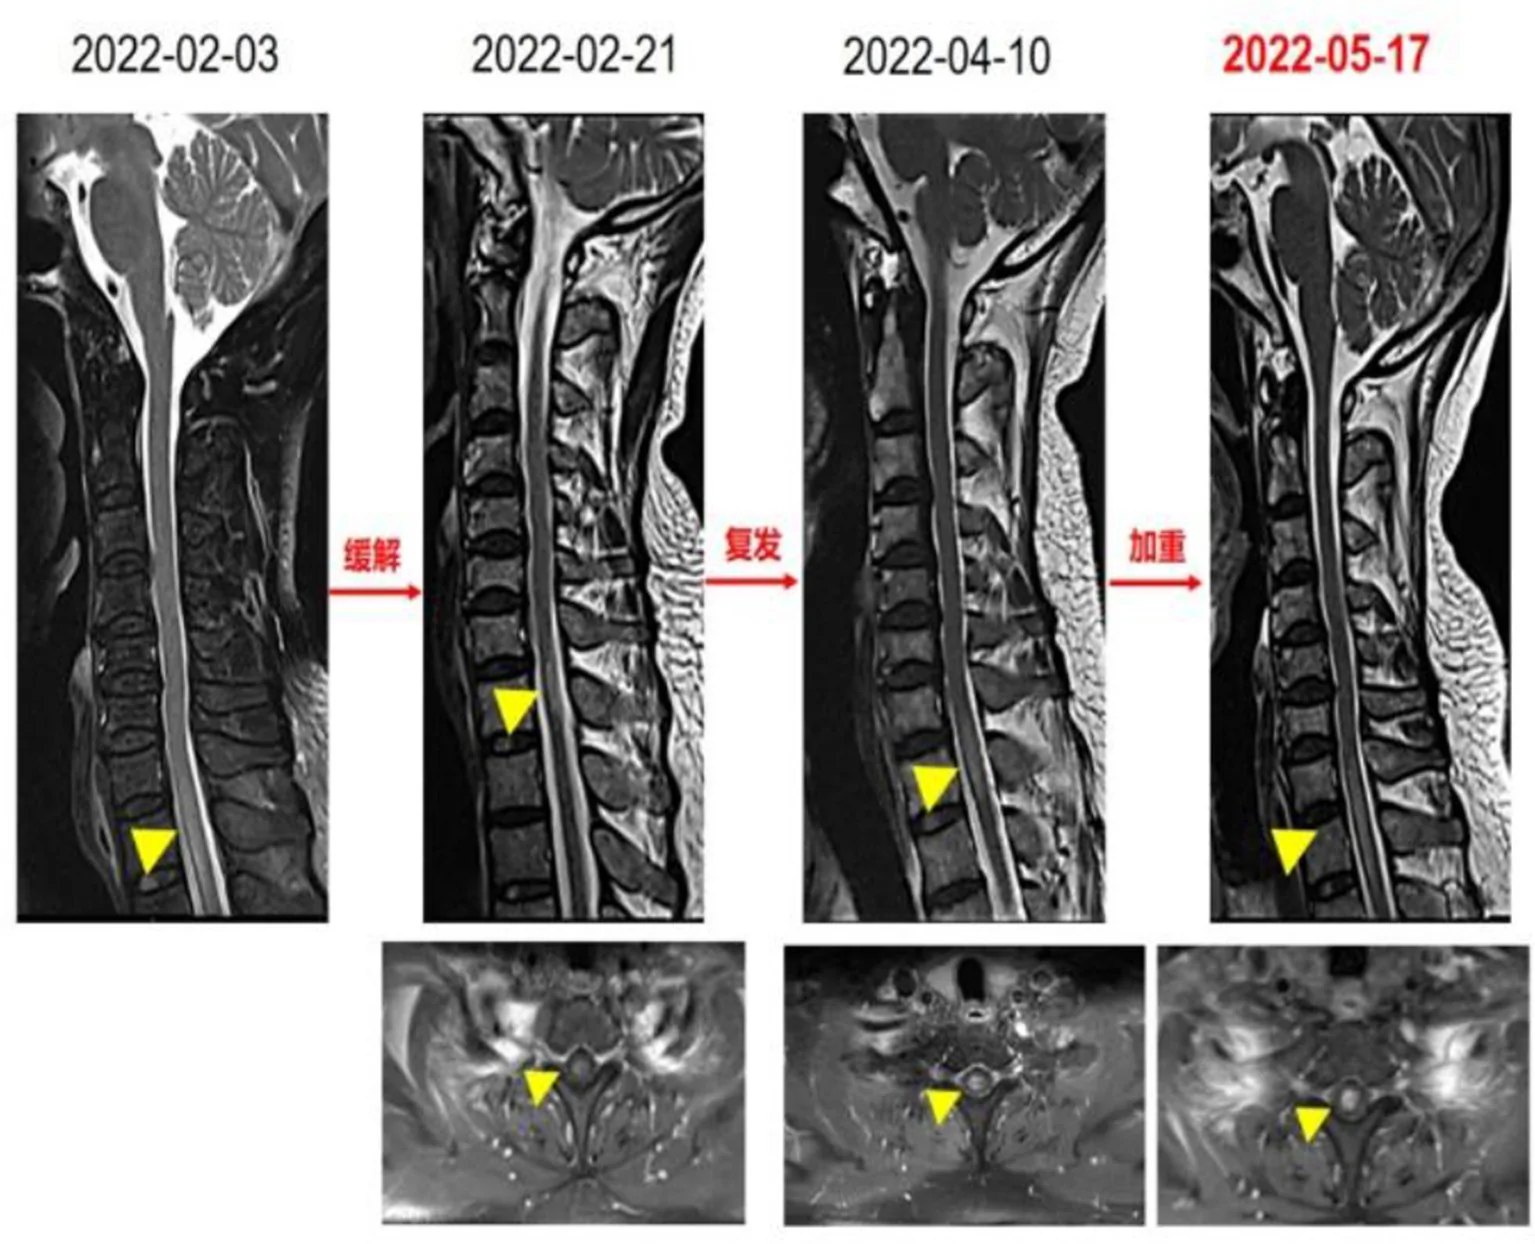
The figure presents cervical and thoracic MR images demonstrating abnormal spinal cord signal at the C7-T1 level, along with the changes in this signal over multiple treatment episodes.
